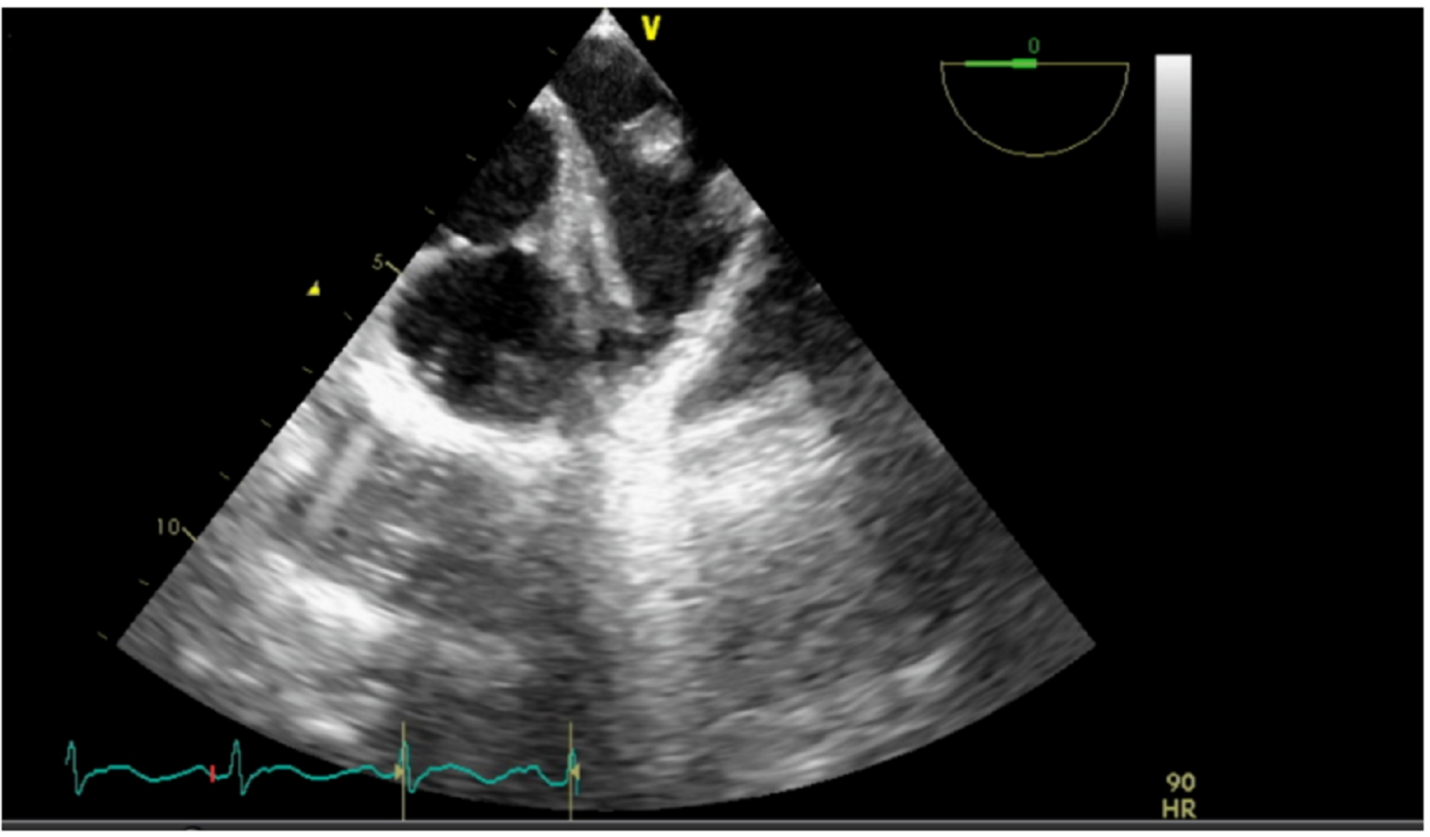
Transesophageal echocardiogram without vegetation
